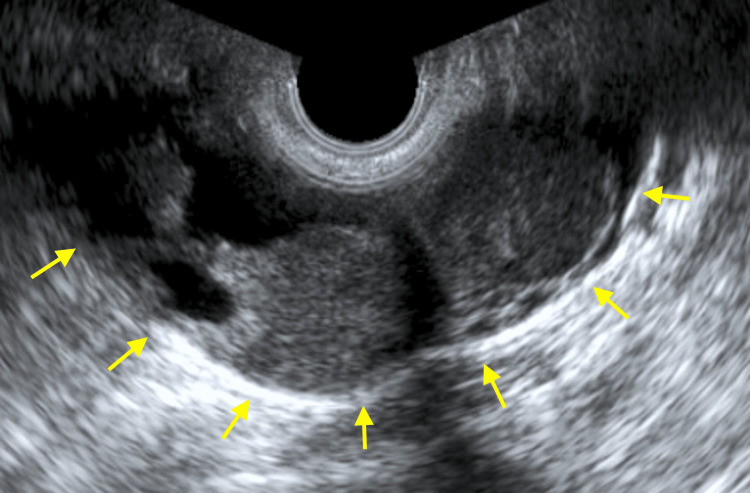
Ultrasound findings. Enlarged left ovary and internal solid component observed on transvaginal ultrasound.
